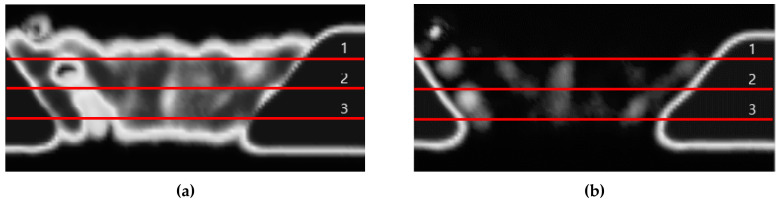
Ultrasonic images by (a) the first back-surface echo and (b) second back-surface echo with three horizontal lines divided into four equal intervals.
